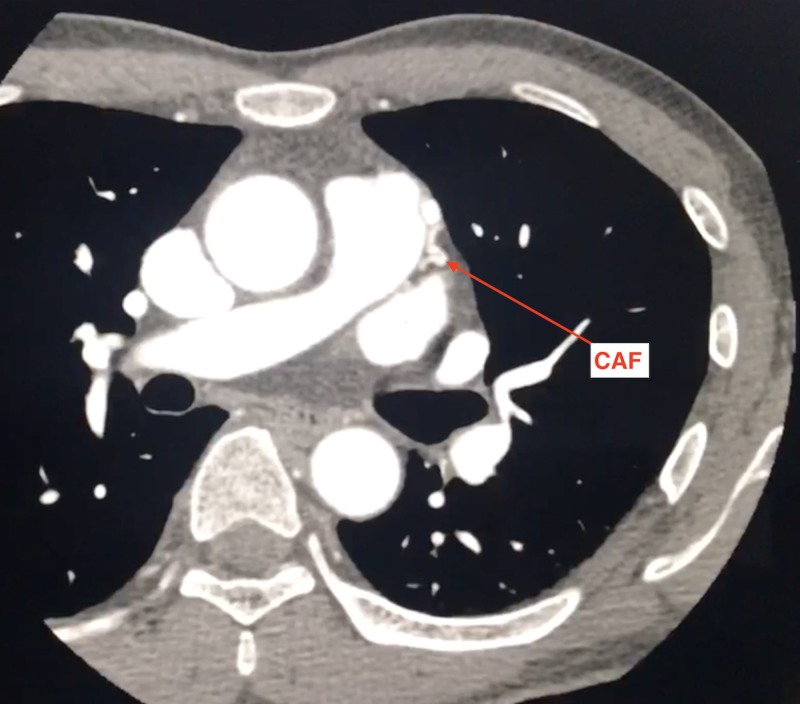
Axial CTA: coronary artery fistula seen as tortuous vessels with aneurysmal dilatation along the left lateral aspect of PA trunk CTA, computed tomography angiography; PA, pulmonary artery
